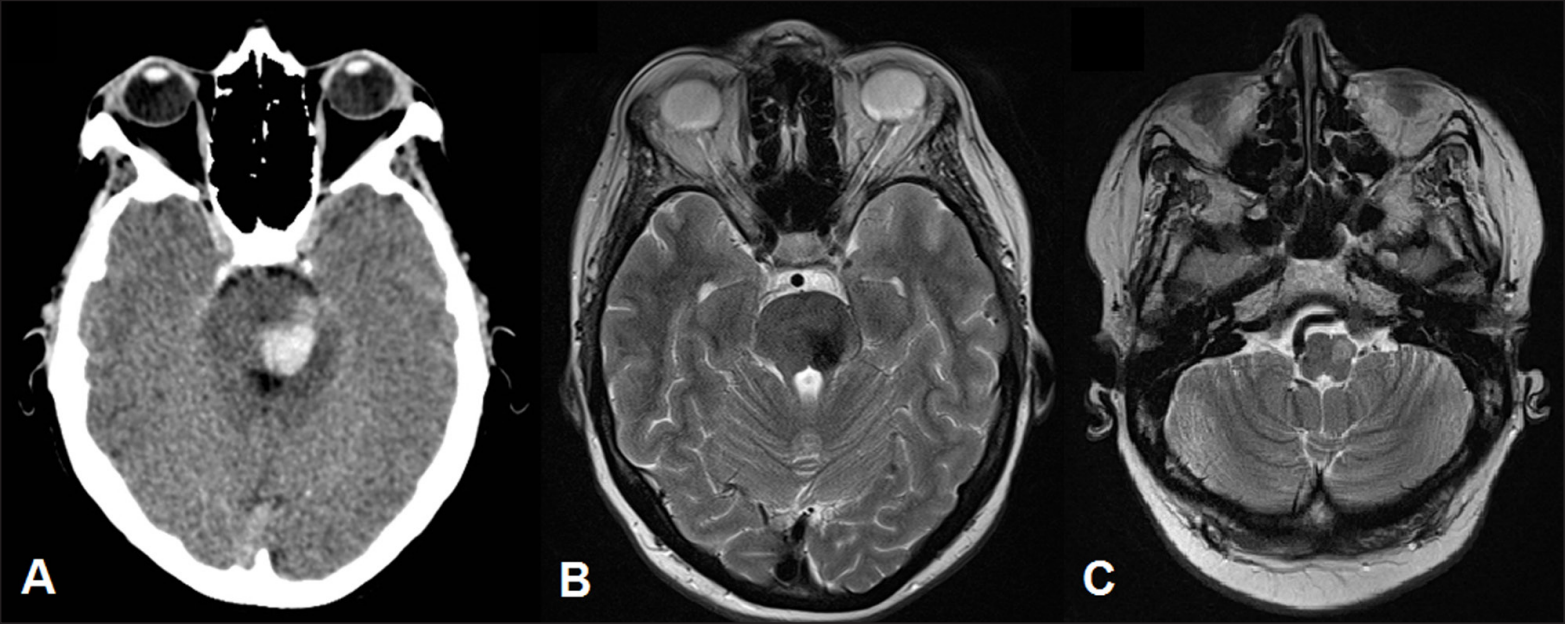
Unenhanced CT shows an acute hemorrhage in the left dorsal pontine tegmentum (A). Follow-up MRI four months later shows residual hemosiderin deposits in the area of the previous hemorrhage (B) as well as a new expansile T2-hyperintense lesion in the left inferior olivary nucleus (C).
